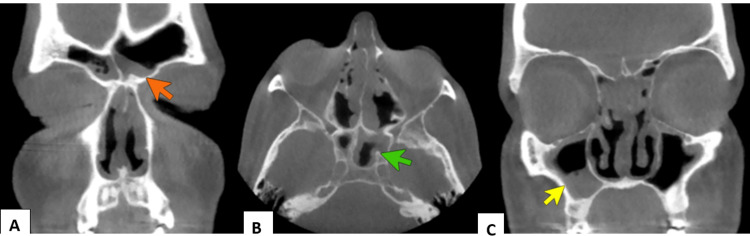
CT of the paranasal sinuses demonstrating chronic inflammation and mucosal thickening of the frontal (orange arrowhead), sphenoid (green arrowhead), and maxillary (yellow arrowhead) sinuses. (A) Coronal view, (B) axial view, and (C) coronal view.
